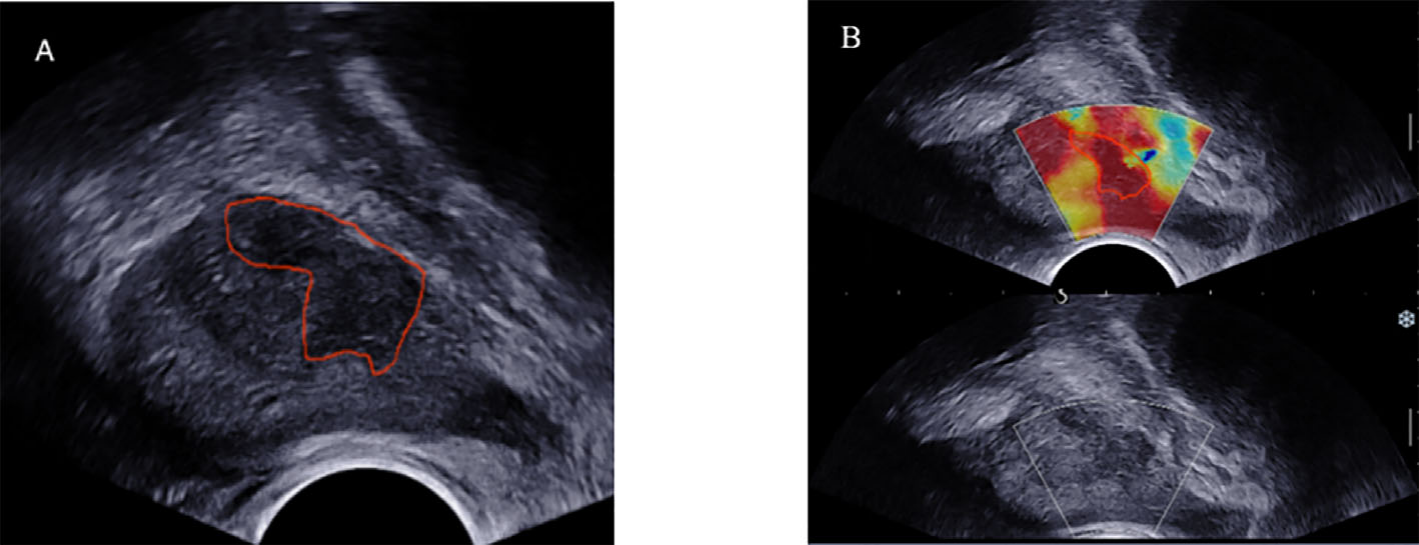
TRUS B-mode ultrasound imaging (A) and SWE imaging (B) from the same position of 74-year-old PCa patient (fPSA, 1.28 ng/ml; tPSA, 7.855 ng/ml; biopsy GS,4 + 3 = 7). ROI (red solid line) was outlined in the B-mode ultrasound and SWE.
